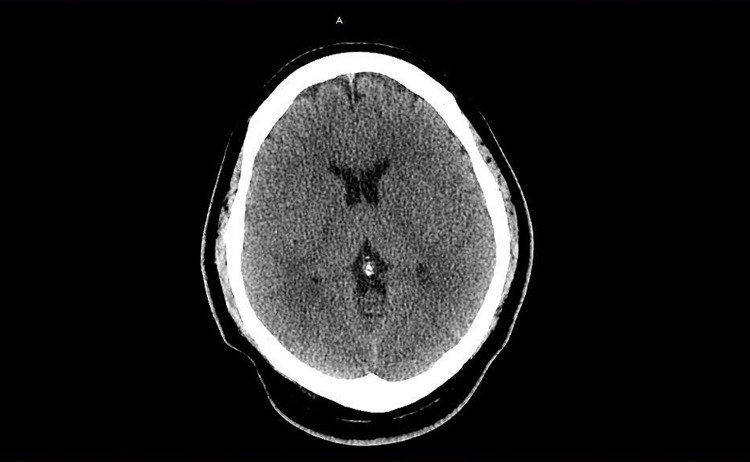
CT head Imaging findings suggestive of early global cerebral edema.
